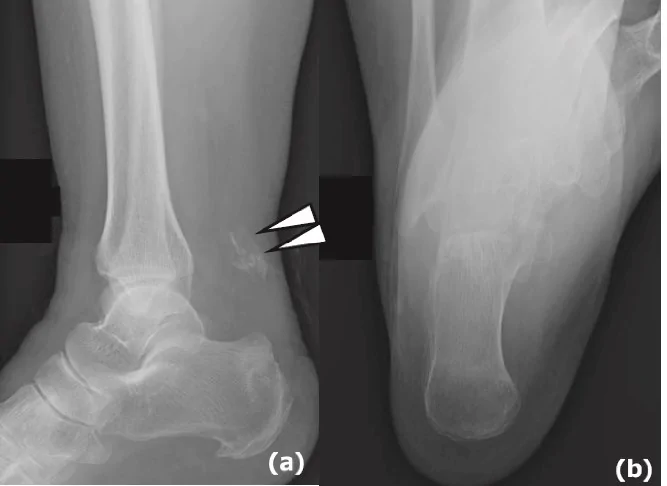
Preoperative radiograph. The sagittal radiograph of the right ankle showing a small avulsion fragment at the posterior calcaneal tuberosity. (a) Sagittal image, (b) axial image.
